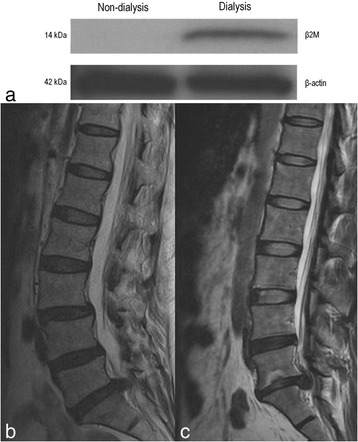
Western blot analysis of the intervertebral disc tissues of one non-dialysis and one dialysis patient. a Western blot analysis of β2M using beta-actin as a loading control. b Sagittal cut MRI image from the non-dialysis patient showed the right L5/S1 herniated nucleus pulposus with grade III degeneration. c Sagittal cut MRI image from the dialysis patient showed left L5/S1 extrusion type herniated nucleus pulposus with Grade IV degeneration
